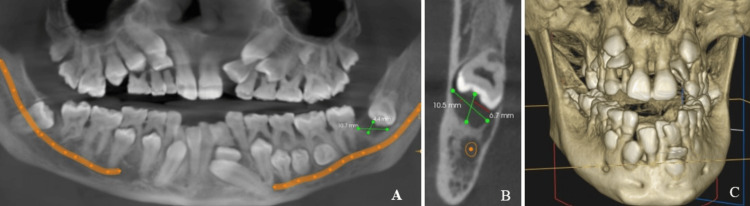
(A) CBCT panoramic mode at a slice thickness of 9.9 mm revealed multiple retained deciduous teeth, multiple impacted permanent teeth, and a 4.4 mm x 10.7 mm circumferential radiolucency encircling the crown of the left mandibular third molar. (B) CBCT coronal section revealed a well-defined circumferential radiolucency measuring about 10.5 mm x 6.7 mm around the crown of the left mandibular third molar. (C) 3D-reconstructed CBCT image also showed multiple retained deciduous teeth and impacted permanent teeth CBCT: cone-beam computed tomography
